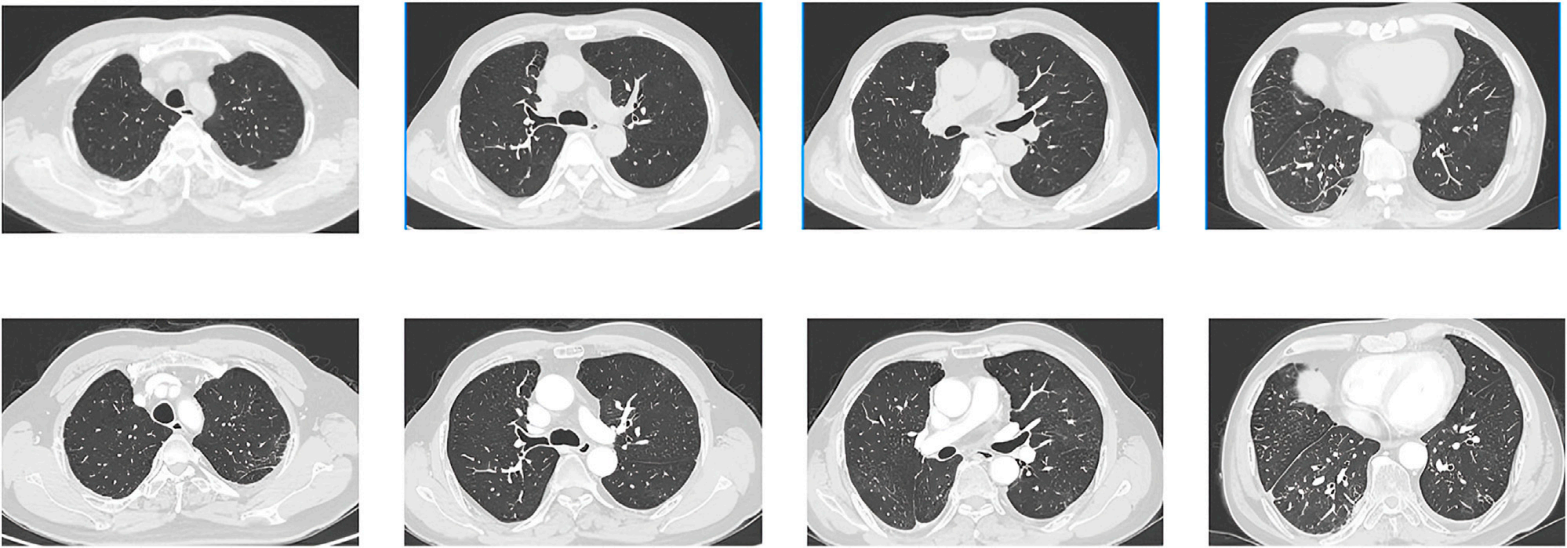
CT images obtained on 2 September 2022(UP) and 10 October 2023 (DOWN). The images showed that the ground-glass opacities, cystic lesions, and patchy consolidations had completely disappeared, and metastatic lesion size continued to be reduced.
